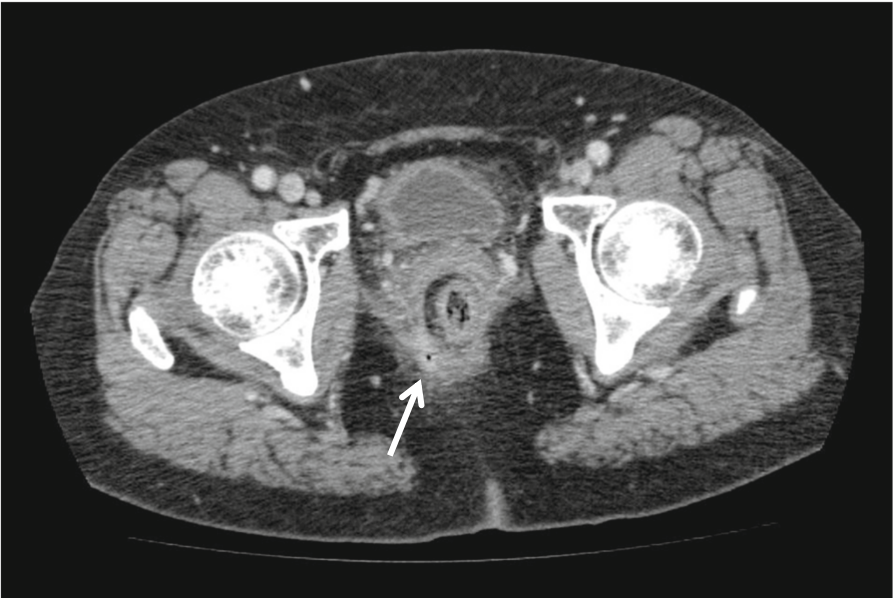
Case 4. CT scan revealed a small amount of extraluminal air existed along an anastomotic-perineal fistula (*arrow*)
